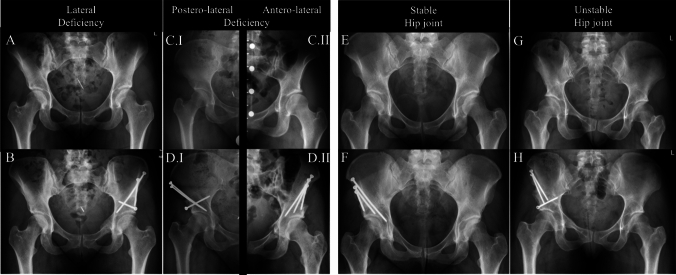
Representative patient cases with different acetabular and hip joint morphologies: pre-(A/C/E/G) and postoperative (B/D/F/H) anterior–posterior pelvic radiographs
